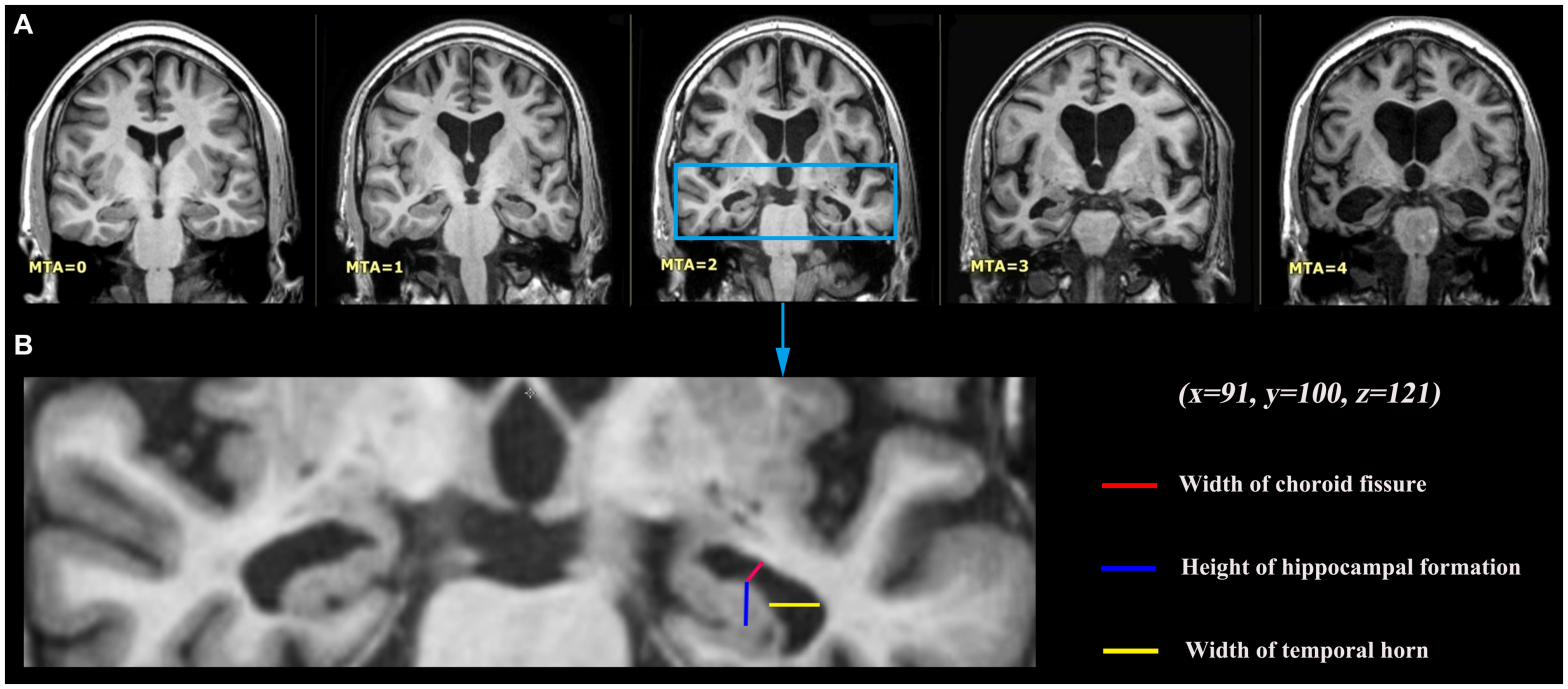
Medial temporal lobe atrophy (MTA) grading criteria. (A) Coronal T1-weighted slices at the level of the hippocampus body of five different study participants with different levels of MTA, 0 no atrophy; 1 widening of the choroid fissure; 2 additional widening of the temporal horn of the lateral ventricle and slightly decreased hippocampal height; 3 moderate loss of hippocampal volume; 4 end-stage increase of all these findings. Those were presented by MRIcron software, with the same coordinate (x = 91, y = 100, z = 121). (B) MTA visual rating is performed on a single coronal slice including hippocampus, with particular attention to the width of the choroid fissure and of the temporal horn, and to the hippocampus height.
